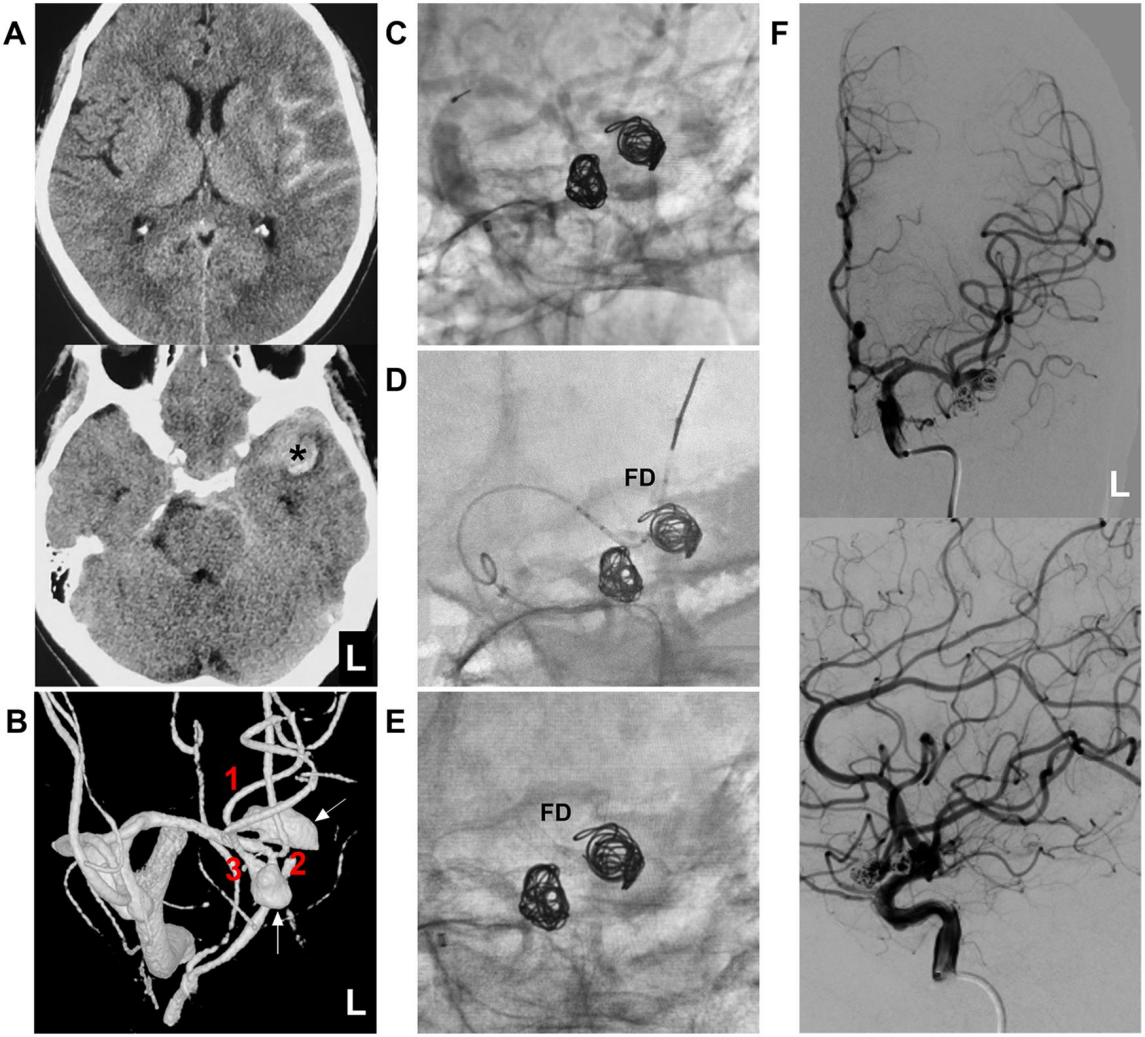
Preoperative CT and EVT course. (A) CT images demonstrate left subarachnoid hemorrhage (SAH) (upper panel) and a small left Sylvian fissure hematoma (asterisk, lower panel), consistent with aneurysm rupture. (B) Three-dimensional DSA reveals the left MCA trifurcation (branches 1-3), with two aneurysms (arrows) located near the origins of the superior and inferior MCA branches. These aneurysms exhibit mirror-like symmetry. (C) Unsubtracted DSA confirms incomplete coiling of the two aneurysms. (D) X-ray demonstrates selective delivery of the FD in the middle MCA branch. (E) X-ray confirms successful FD deployment in the middle MCA branch. (F) DSA images of the left internal carotid artery in lateral (upper panel) and anteroposterior (lower panel) views demonstrate the patency of all three MCA branches post-EVT. CT, computed tomography; DSA, digital subtraction angiography; EVT, endovascular treatment; FD, flow diverter; L, left; MCA, middle cerebral artery; SAH, subarachnoid hemorrhage.
